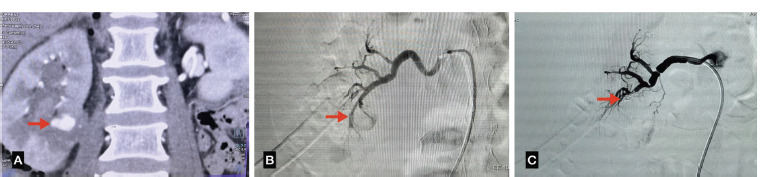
(A) CT Angiography showing a pseudo aneurysm in the right lower interloper renal artery. (B) Diagnostic angiogram showing a pseudo aneurysm in the right lower polar artery. (C) Post embolisation image showing a coil in the lobar artery causing occlusion of the pseudo aneurysm.
